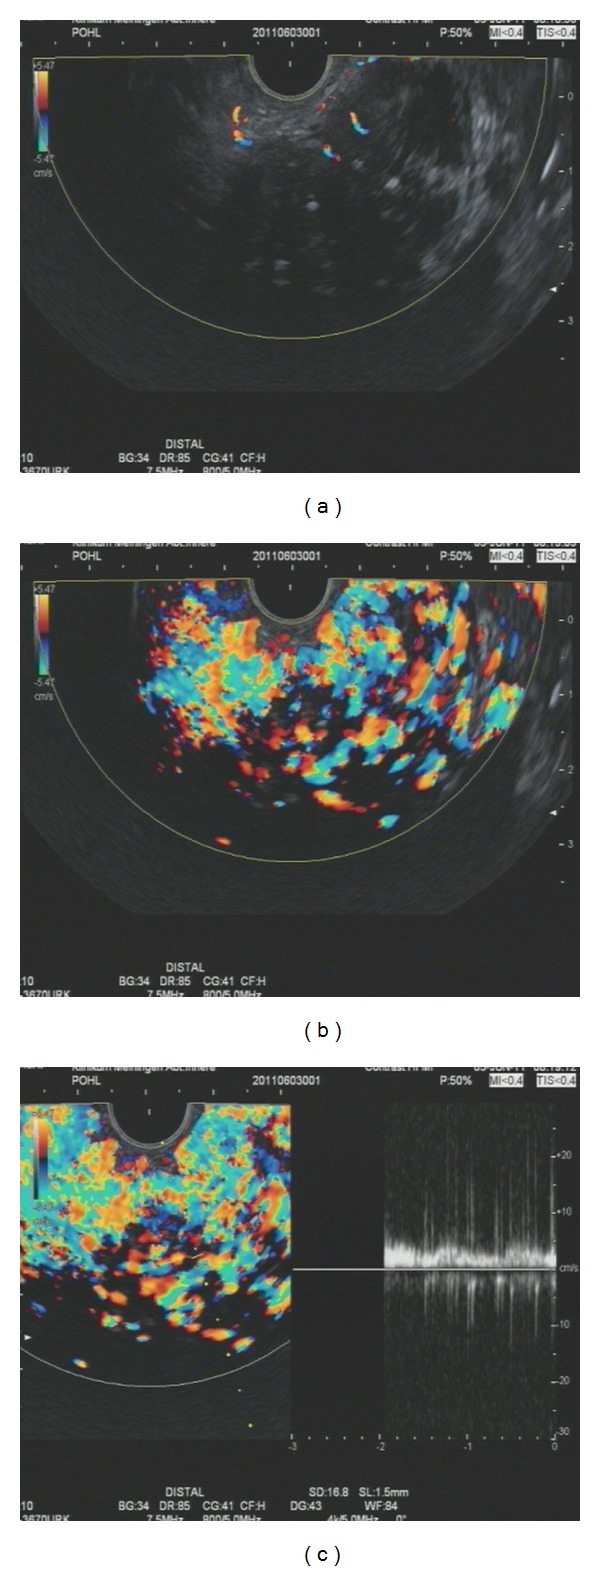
Contrast-enhanced high mechanical endoscopic ultrasound in a patient with chronic pancreatitis: (a) lesion before contrast enhancer injection the lesions is visible within the colour doppler window as a nearly black area; (b) Lesion after contrast enhancer influx (4.5 mL Sonovue) with a visible netlike vessel system the rich vessel system is visible mostly on the right side of the picture with different colours; (c) pw-Doppler analysis of the vessels with a clear venous signal; on the right half of the picture a laminar flow is displayed as a nearly flat white bark.
